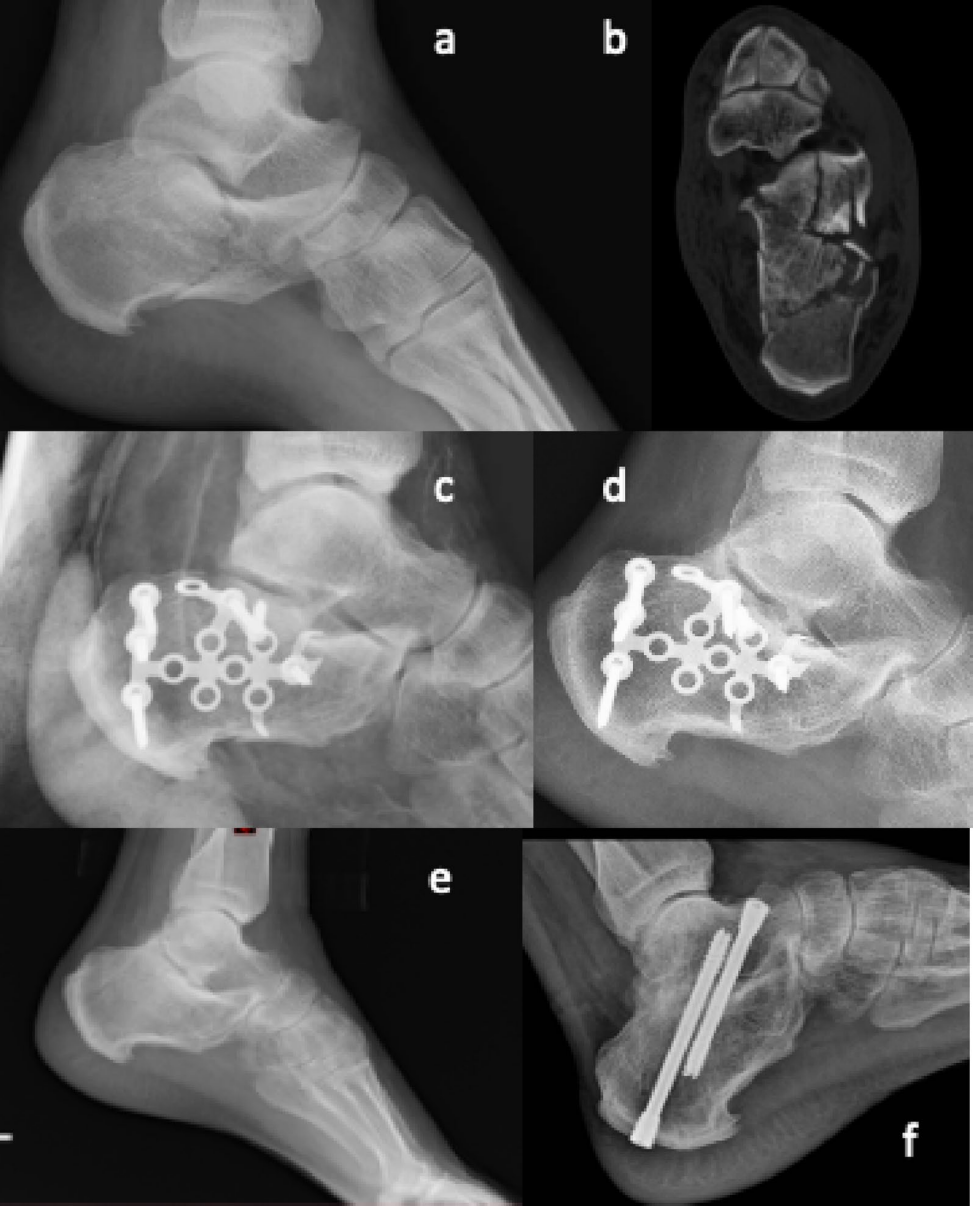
Clinical case of calcaneal fracture about our Institute (Department of Ageing, Neurosciences, Head-Neck and Orthopedics Sciences, Orthopedics and Trauma Surgery Unit, Fondazione Policlinico Universitario Agostino Gemelli IRCCS). a Lateral X-ray view of calcaneal fracture; b preoperative axial view of CT scan; c postoperative lateral view of ORIF for calcaneal fracture; d 1-month postoperative X-ray control; e secondary subtalar arthritis 3 years after surgical treatment; f subtalar arthrodesis made by two cannulated partially treated screws, postoperative X-ray control, 5 years after first treatment
